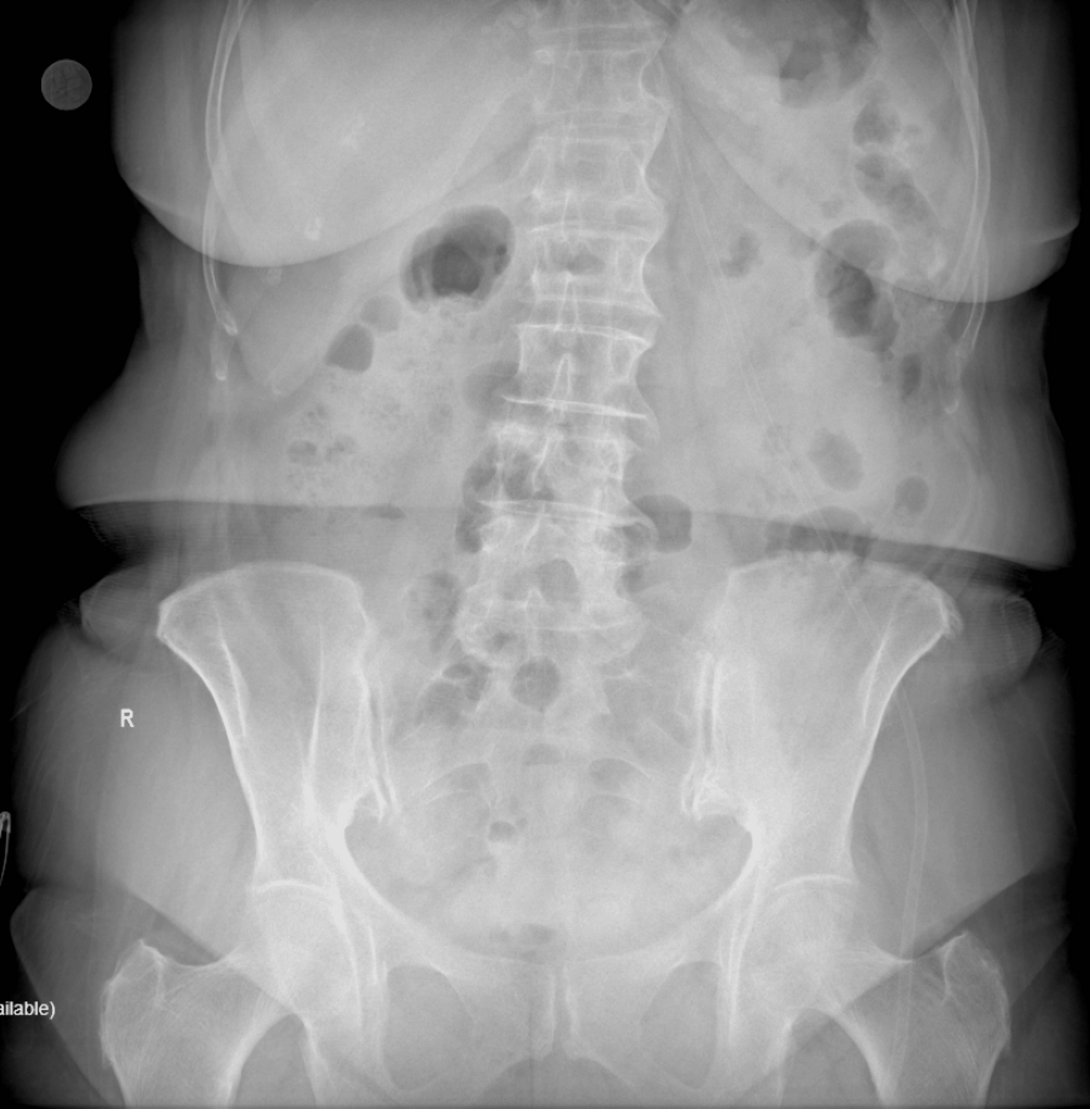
An abdominal X-ray upon presentation in the emergency department showed no dilated bowel loops nor air-fluid levels present.
